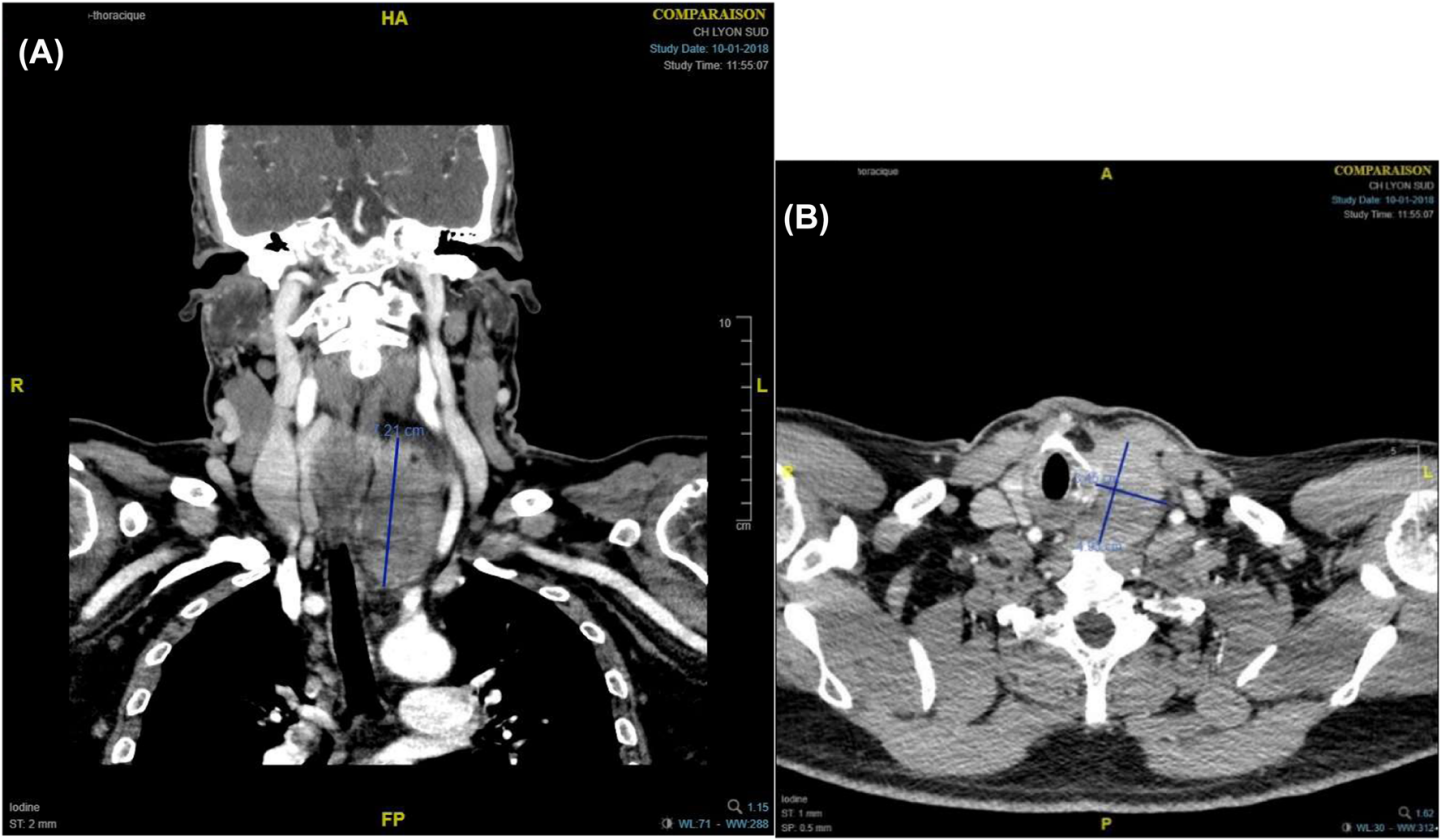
Second CT scan (with contrast). (A) Coronal view. (B) axial view.
